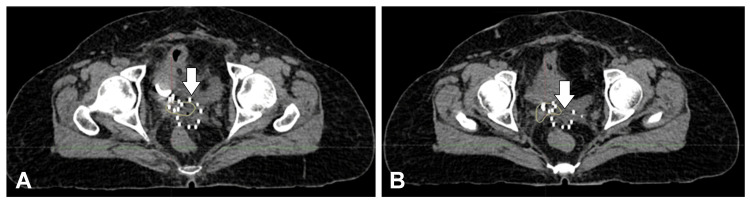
(A) An image of the interstitial brachytherapy application on the patient using Kelowna applicator and interstitial needles (white arrow). (B) An image of the planning CT scan for interstitial brachytherapy showing the interstitial needles (white arrow) CT: computed tomography
